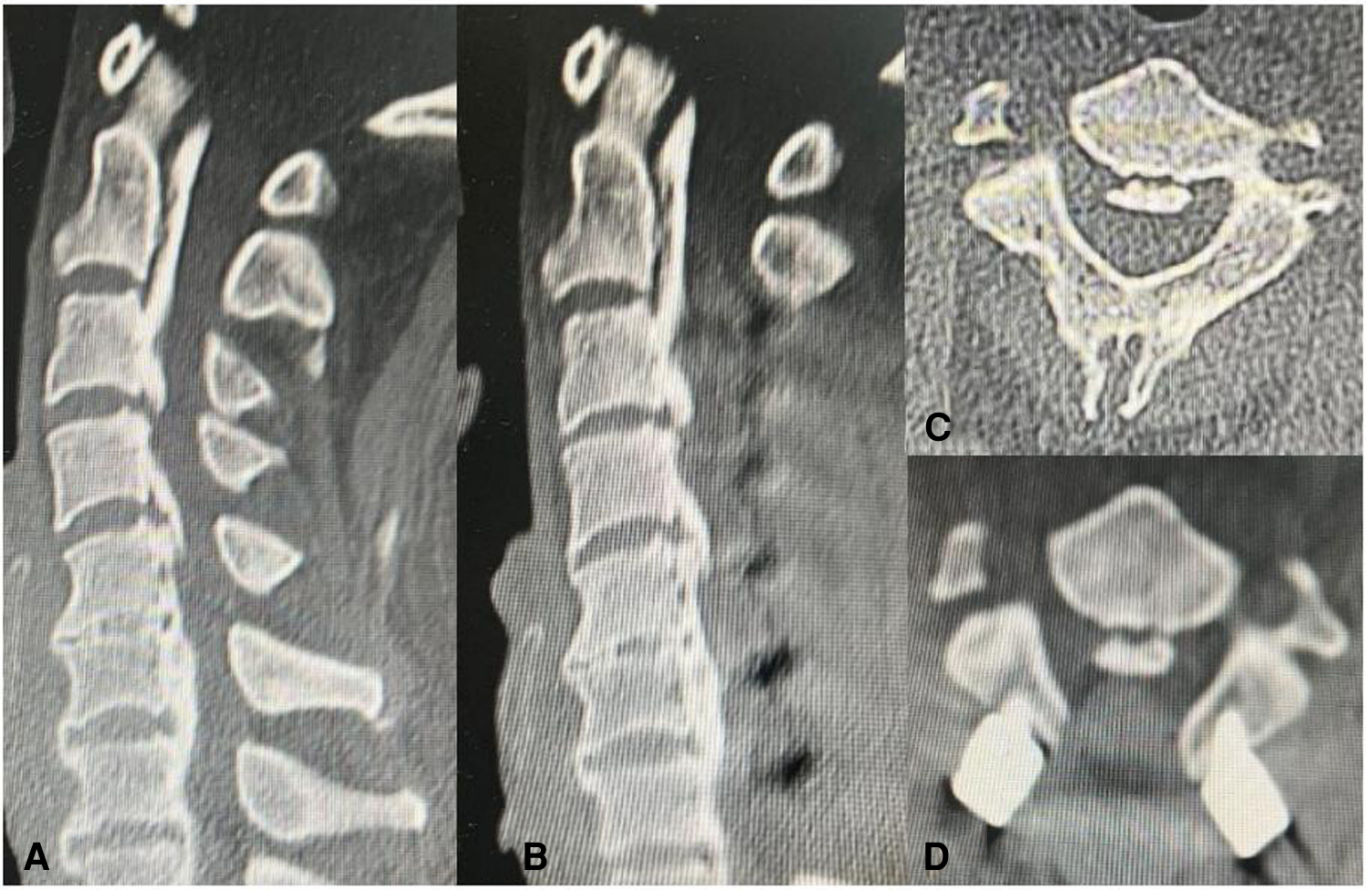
CT scans showing the extent of bone removed by C2 partial laminectomy (C2-PL). (A,C) showing the CT image of the cervical spine before the surgery. (B,D) showing the CT image of the cervical spine after the surgery.
